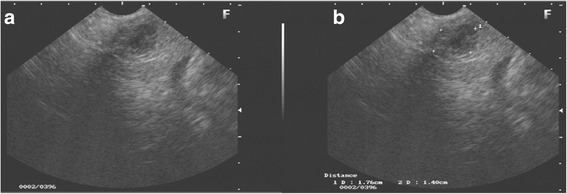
Endoscopic ultrasound view of the solid cystic lesion in the pancreas. a Rounded lesion, with well-defined contours, sharp and precise borders, with solid and cystic areas. b Solid lesion with cystic components measuring 1.7 × 1.4 cm
